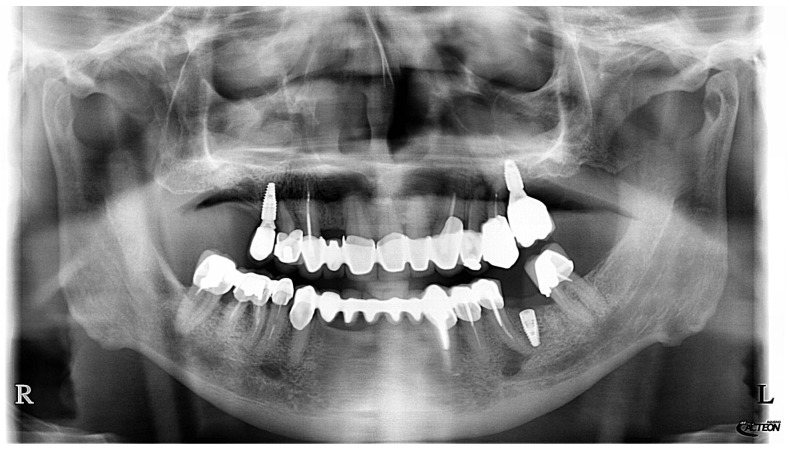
A visualization of a periapical lesion in a panoramic radiograph. The lesion appears as a darker radiolucent area (indicating decreased bone density) located at the apex of the tooth root. This darker appearance is due to the bone resorption and inflammatory process which is characteristic of periapical periodontitis.
